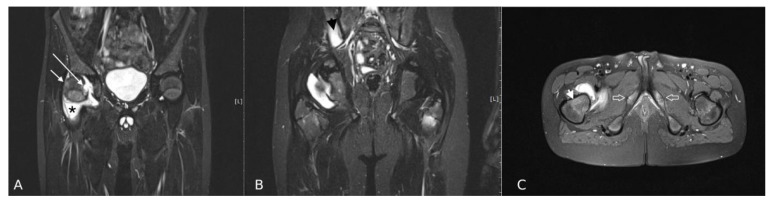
MRI of the hips in a 10-year-old boy with JIA: (A,B) Coronal T2 TIRM, (C) Axial T2 TIRM images. Right hip: joint effusion (stage 3), synovitis (stage 1) (asterisk), BME in femoral head (stage 1) (white short arrow), BME in the neck of femur (stage 2) (arrowhead on “C”), erosion in the femoral head (white long arrow). Left hip: BME in the femoral neck (stage 2). Right sided sacroiliitis (arrowhead on “B”). Involvement of ischiopubic synchondrosis bilaterally (empty arrows on “C”).
